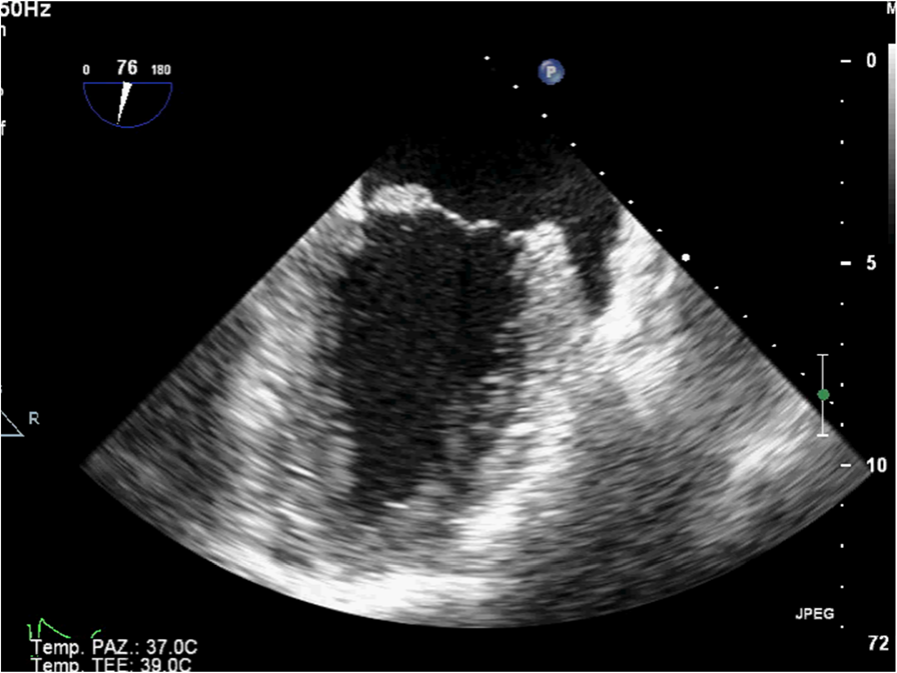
Transesophageal echocardiogram. The intercommissural view (76°) shows a mobile nodular mass, measuring 1 × 1 cm, on the anterior leaflet of the mitral valve
